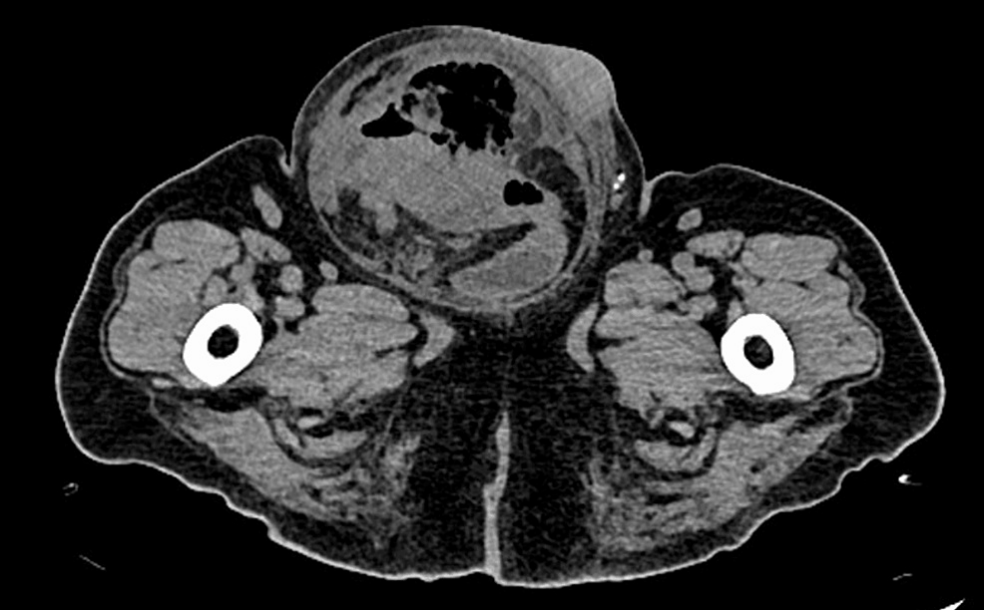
CT scan showing a right inguinoscrotal hernia with a mass within the bowel lumen and free air inside the hernia CT: computed tomography
